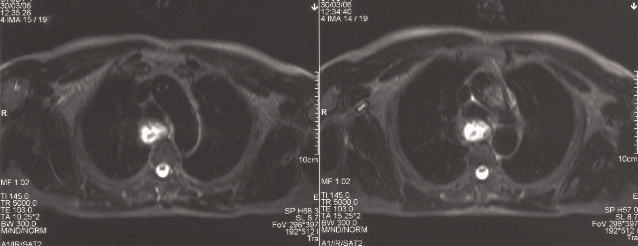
MRI T2-W axial image: the esophageal tumor appears non homogenous, with sharp margins.
